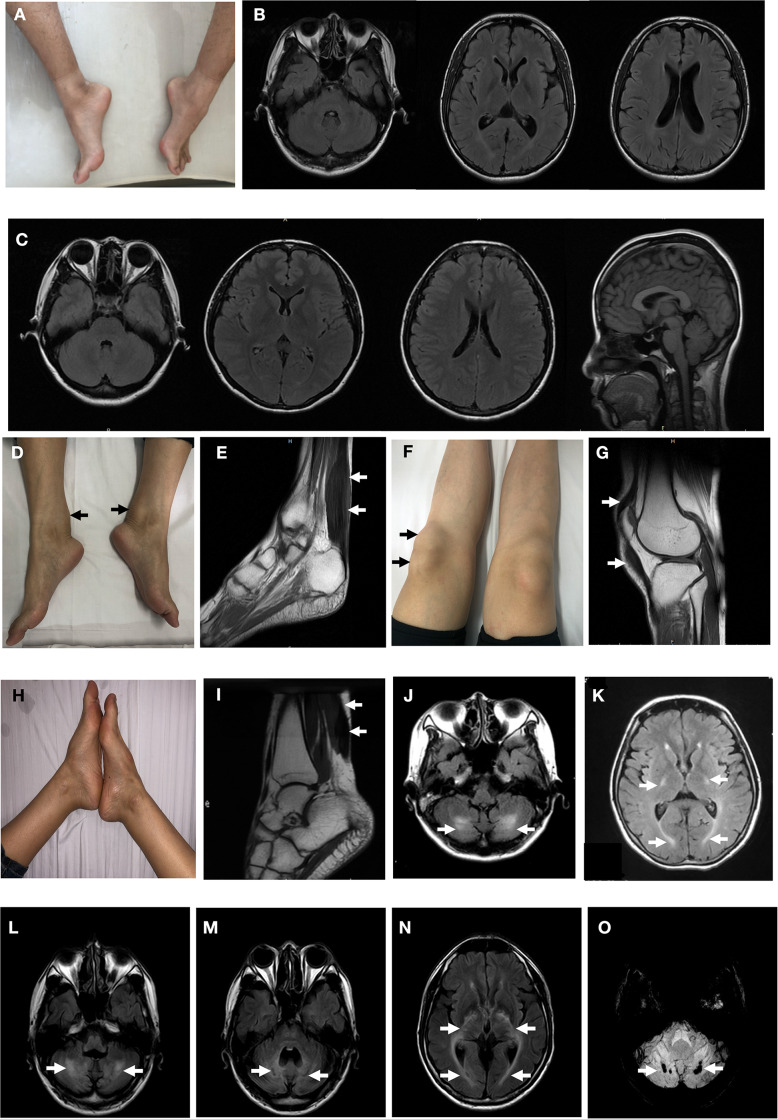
Clinical findings of the probands. Pes cavus deformity of Patient T4550 (A). Brain magnetic resonance MR) of fluid attenuated inversion recovery (FLAIR) images were normal with Patient T4550 and Patient T4979 (B,C). Thickened bilateral Achilles xanthoma and ligamentum patellae of Patient T4979 (D,F). Xanthoma of the Achilles tendon on T2-weighed MRI (arrow) (E). Thickened ligamentum patellae on T2-weighed MRI (arrow) (G). Achilles tendon xanthoma located at right side and bilateral pes cavus of Patient T5200. (H) MRI demonstrated fusiform enlargement involving the Achilles tendons of Patient T5200 (arrow) (I). FLAIR MRI of Patient T5200’s brain showed bilateral high-intensity areas in the dentate nuclei (J) and periventricular white matter (K) (arrow). Patient T5300’s brain MR imaging showed hyperintensity lesions (L) and hypointensities (M) located at the dentate nuclei (arrow), and periventricular white matter hyperintensities (N). (O) Susceptibility-weighted imaging. Multiple microbleeds are seen in the bilateral dentate nuclei of Patient T5300.
